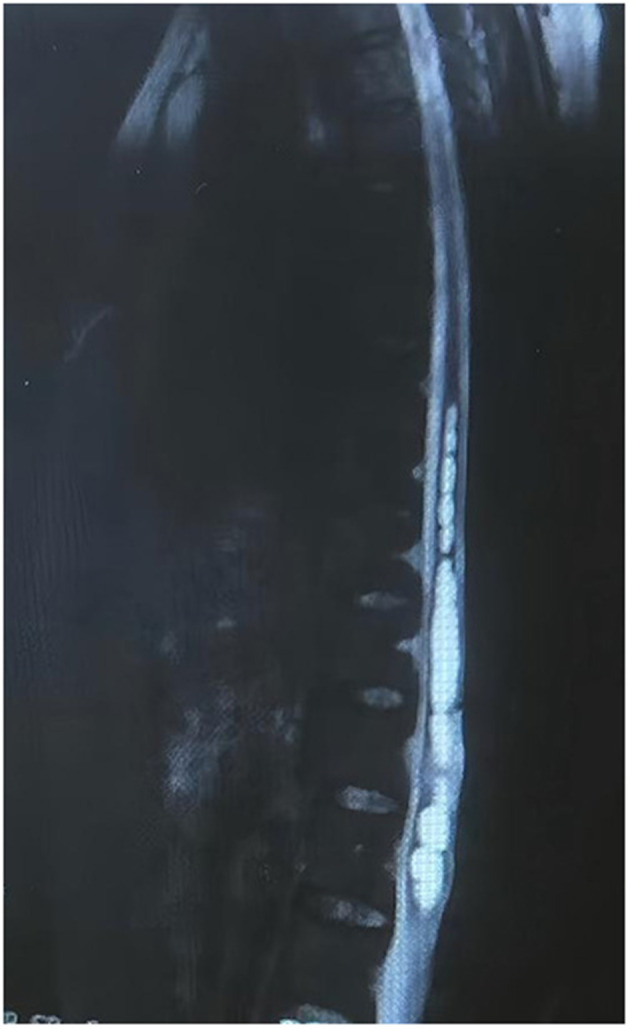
Spinal MRI provided by the patient, revealing an intradural cysticercal lesion.
